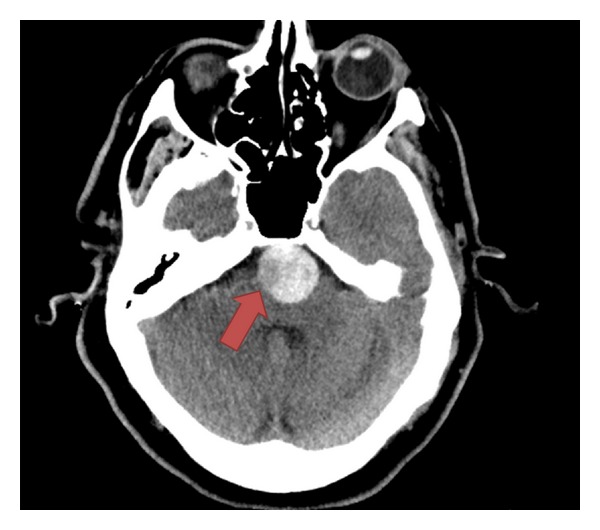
CT head without contrast: 25 mm basilar artery aneurysm with partial thrombosis and no evidence of rupture.
